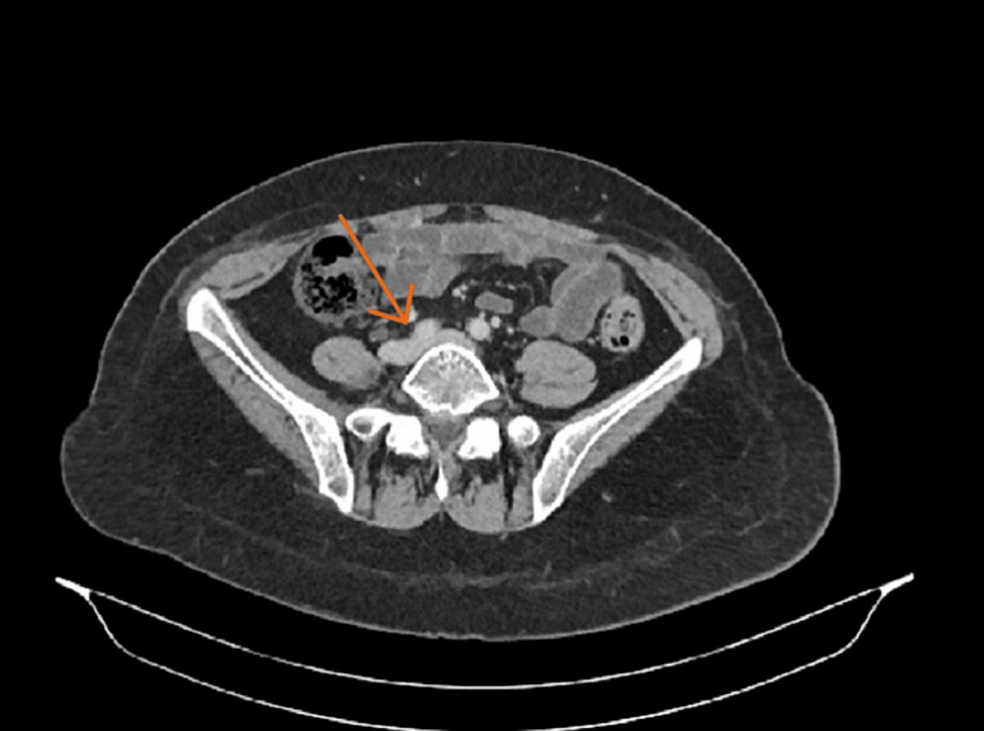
Preoperative CT scan showing a compression of the LCIV by the RCIA LCIV, left common iliac vein; RCIA, right common iliac artery
